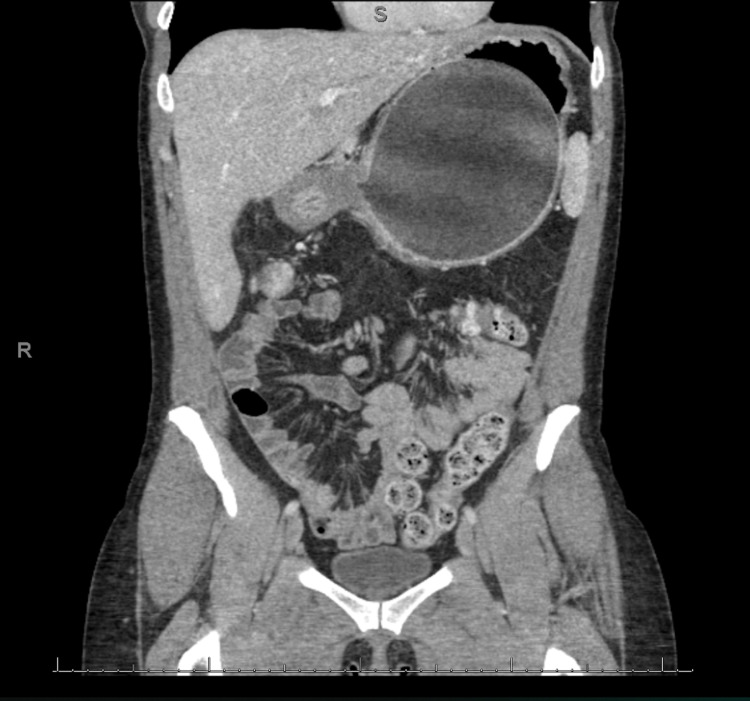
Coronal CT image showing enlarged IGB with stretched gastric wall. IGB, intragastric balloon
